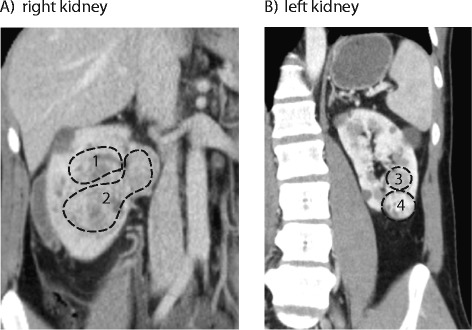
Multi-focal renal cell carcinoma tumors in a patient with VHL disease. Coronal sections from computed tomography scans show the spatial orientation of the tumors within the kidneys. (A) A macroscopically single tumor in the right kidney, but defined by exome sequencing as two tumors (one and two). (B) Two separate tumors in the left kidney, tumors three and four. Cysts typical of VHL disease are also present in both kidneys.
